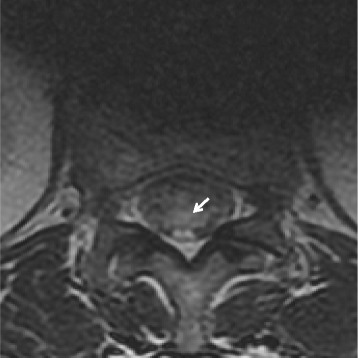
MRI shows a hyper-intense signal at the lower end of the conus medullaris in the T2-weighted image. The *arrow* points to the area of the ischemic lesion, which is white in contrast to normal nerve tissue
